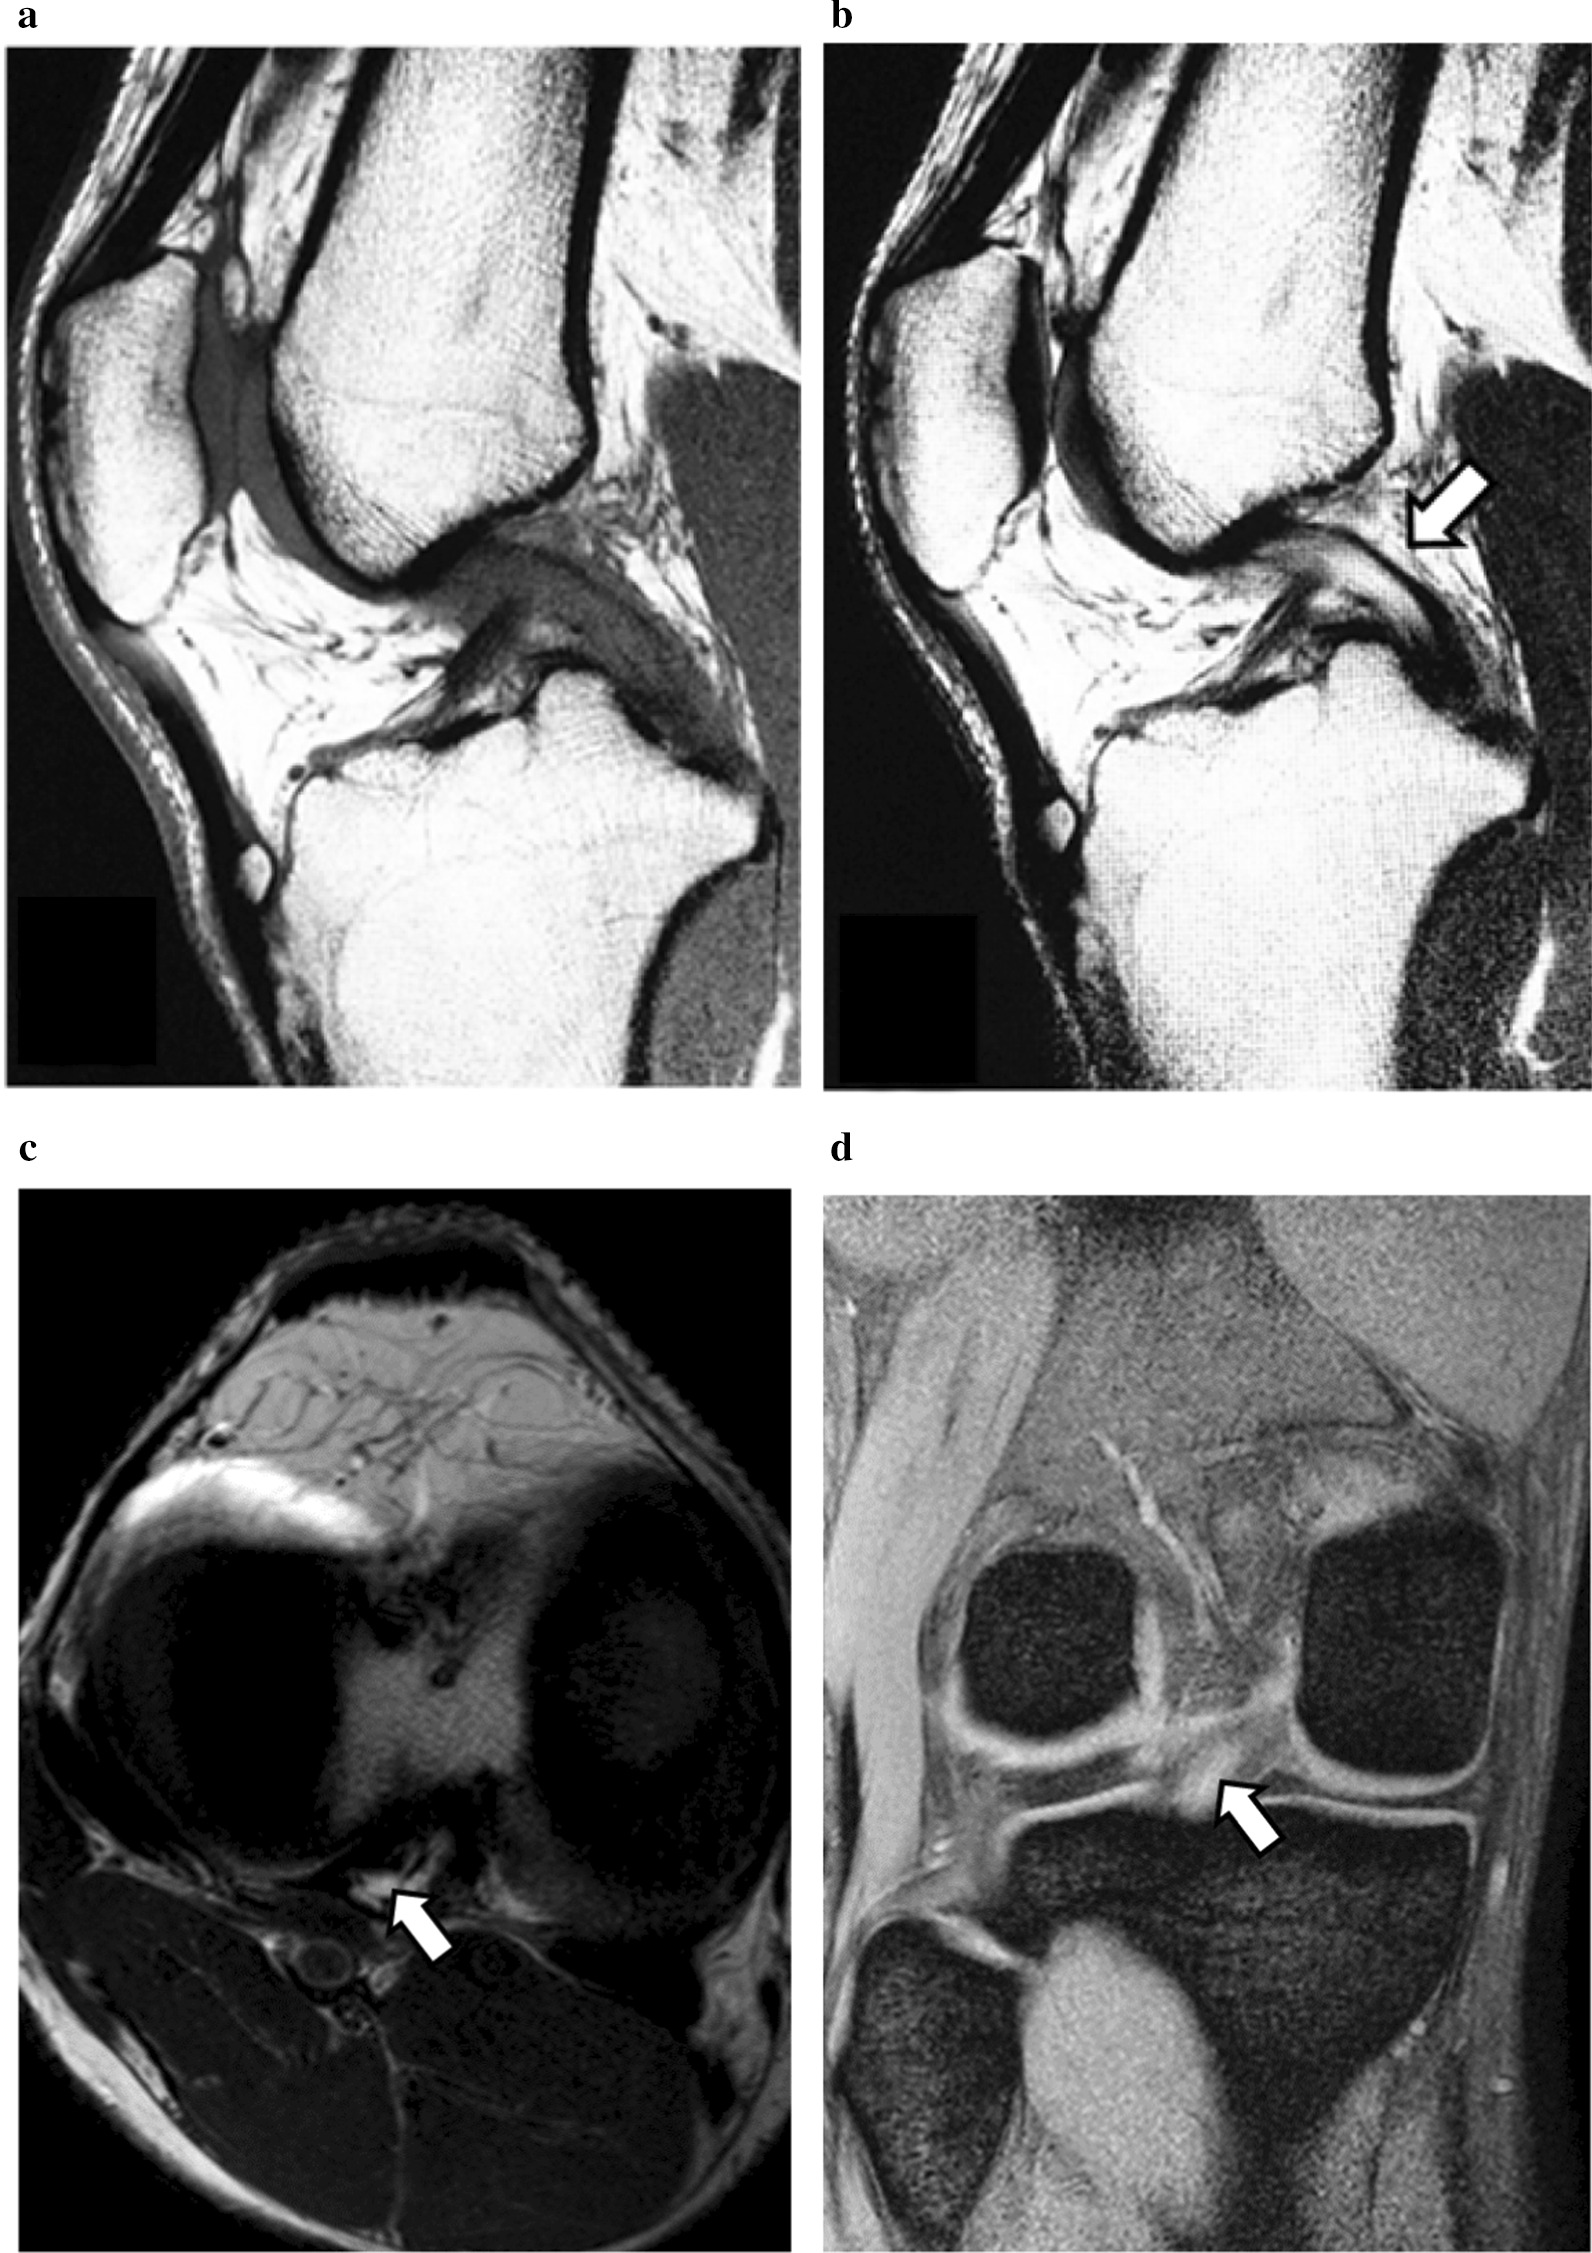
Preoperative MRI. a Sagittal T1-weighted image of the PCL showing relative increased signal within ligament consistent with diffuse mucoid degeneration. b Longitudinal increased signal intensity area within the PCL on sagittal T2-weighed images with an adjacent well-defined rim of hypointense PCL fibers (white arrow). c Axial and d coronal T2-weighed images of the PCL showing high signal intensity area within the PCL (white arrow)
